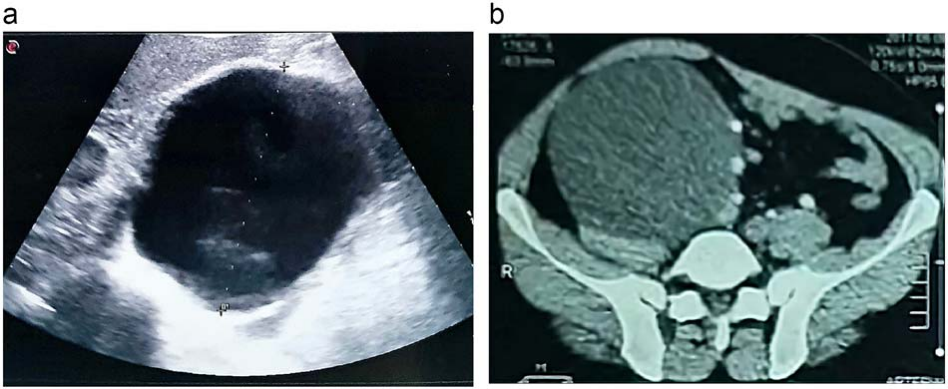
a. An US image showing a large cystic mass in the right abdomen. b. A CT scan of the abdomen showing a cystic mass of 17 × 11 × 10 cm with a fat-fluid level.
